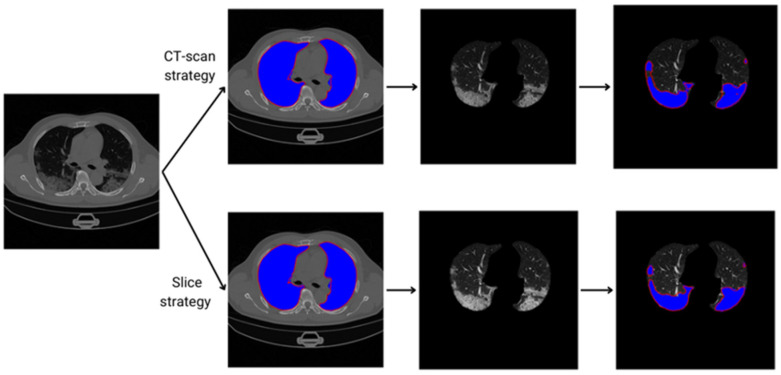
The first row shows the lung and COVID-19 images segmented with U-Net trained with the CT scan strategy. The second row shows the lung and COVID-19 images segmented with U-Net trained with the slice strategy. Radiologist segmentation (Dataset 1) is shown in blue, whereas automatic segmentation is shown in red.
